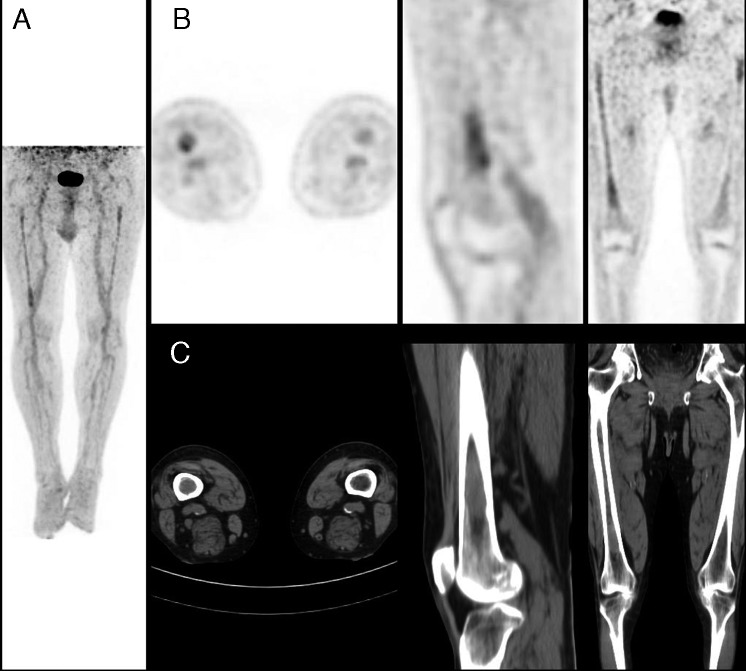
^18^FDG-PET/CT of whole body and lower extremities. (A) Maximum intensity projection image of lower extremities. (B) PET slices: pathological uptake in the distal region of the right femur (SUVmax: 3.6). (C) CT slices: high-density 4.5 cm intramedullary lesion in the right femoral diaphysis.
